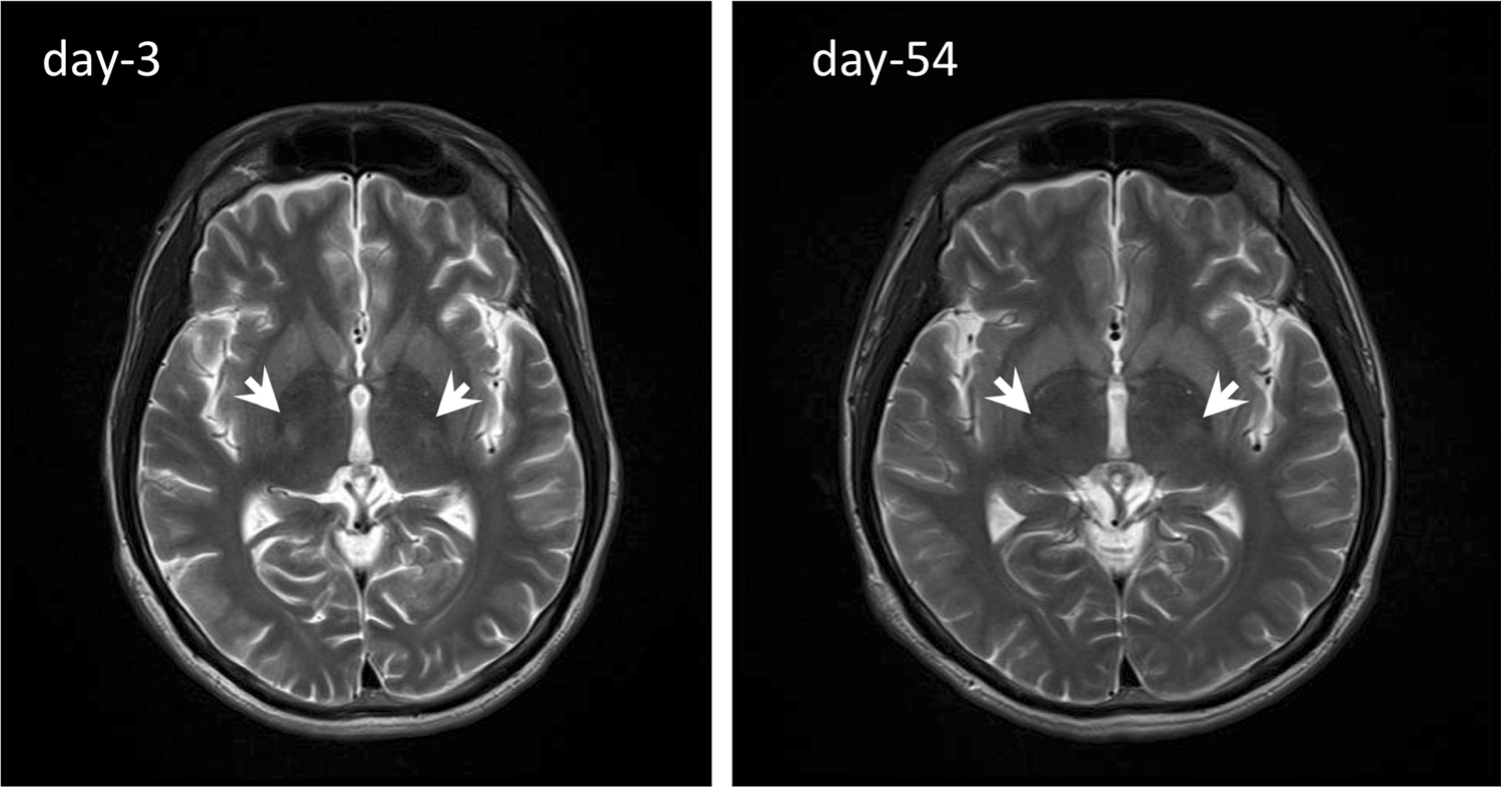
Brain MRI study (T2-weighted image) on hospital day-5 and day-54. *White arrowheads* indicate high-intensity signals in the ventral posterolateral nucleus (VPL)
